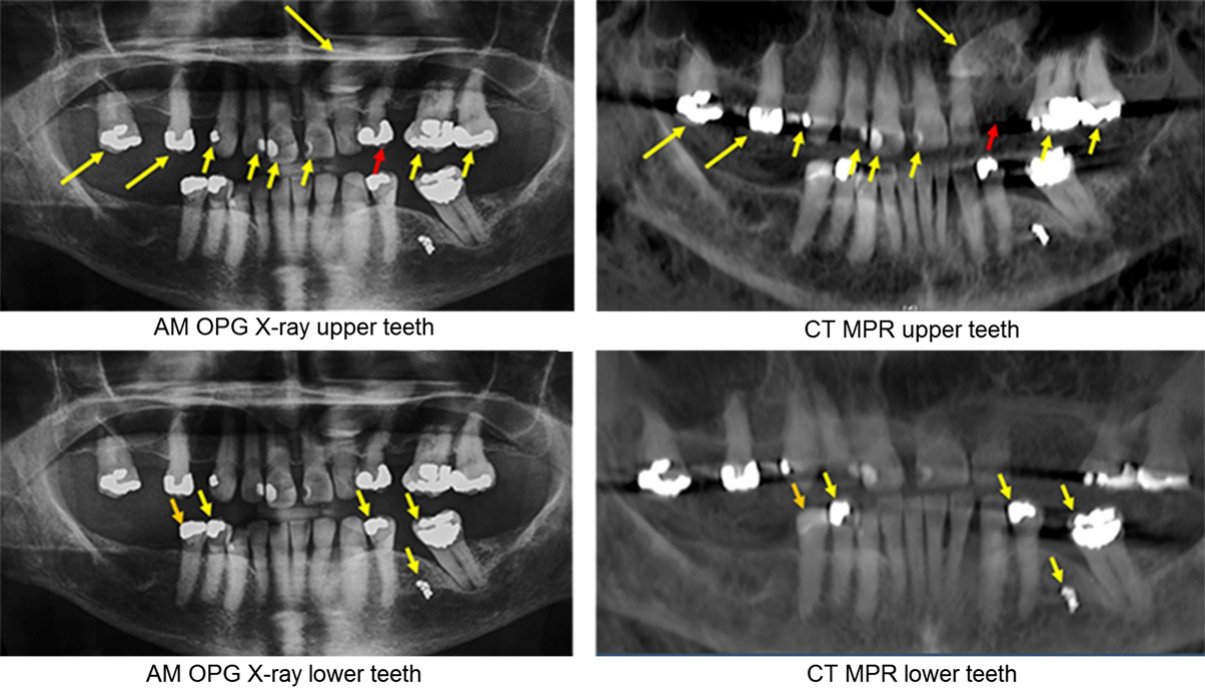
Comparison between antemortem (AM) orthopantomogram (OPG) X-ray of a missing person and multiplanar reconstructions (MPR) of CT data of the upper and lower jaws of a victim. Corresponding features are indicated by yellow arrows. The red arrow in the upper jaw indicates a tooth that was extracted before death and the orange arrow in the lower jaw indicates a filling that was replaced. Both are accounted for in the written dental record. (Image courtesy Health Support Queensland Forensic and Scientific Services (HSQ FSS) and the Queensland State Coroner, with permission).
